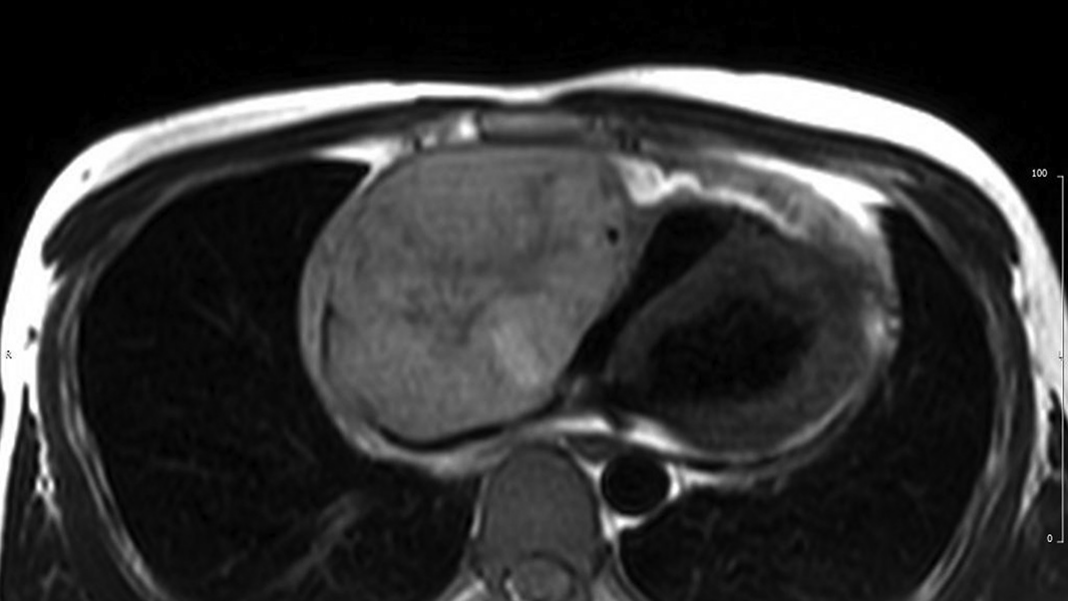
T_2_-Weighted Cardiac Magnetic Resonance Image Showing the Right Atrial Mass
